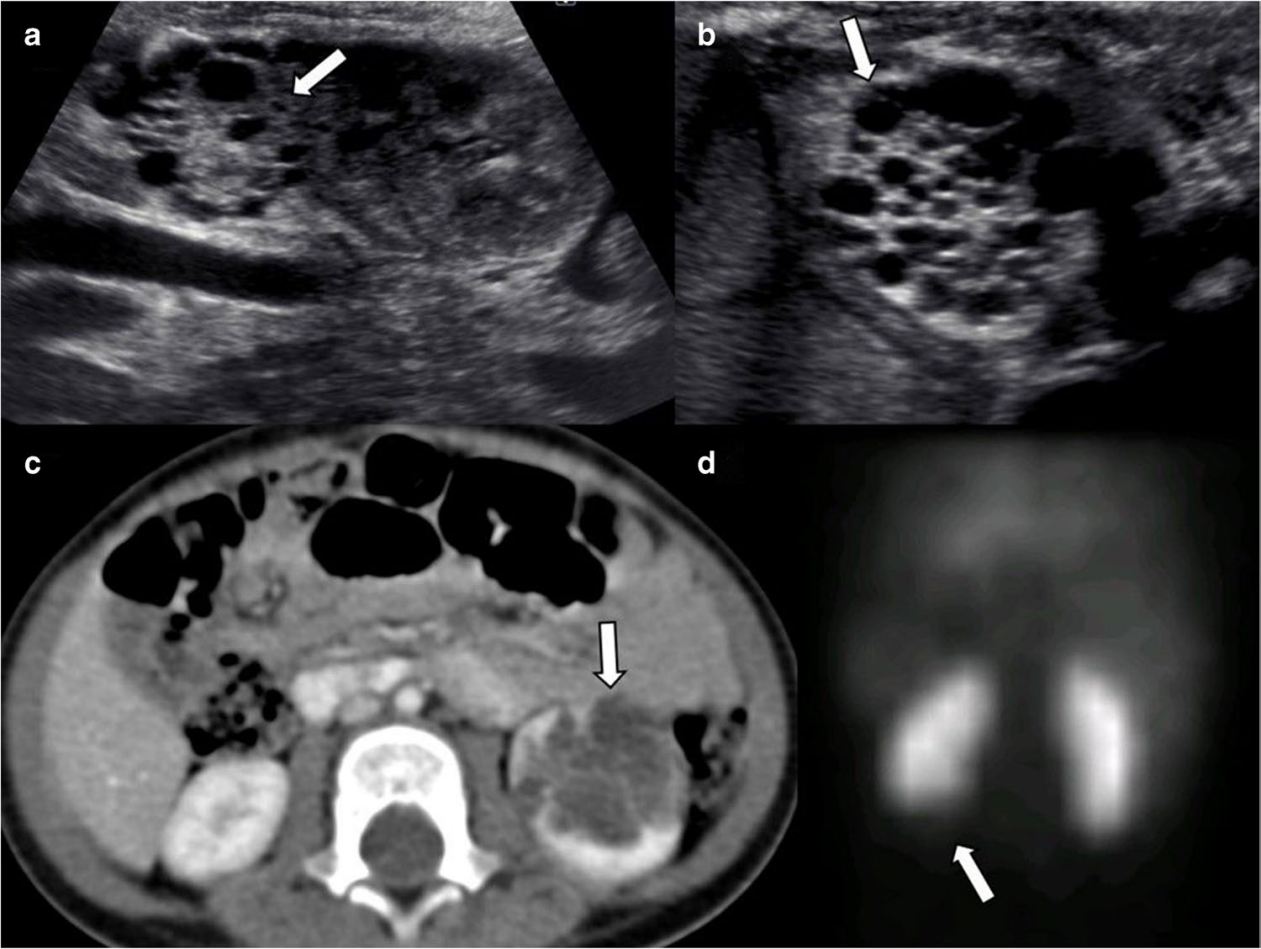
Segmental multicystic dysplastic kidney. Two girls aged 3 months (a, b) and 15 months (c, d) presented to the hospital with recurrent urinary tract infections. a, b Longitudinal (a) and axial (b) grayscale ultrasound images show a lesion (*arrows*) composed of non-communicating small cysts and echogenic stroma in the upper pole of the left kidney. c Axial contrast-enhanced computed tomography image reveals a multilocular cystic lesion composed of cysts of various sizes (*arrow*) in the lower pole of the left kidney. d Dimercaptosuccinic acid scintigraphy shows an absence of radiotracer uptake at the corresponding level (*arrow*). These lesions remained stable during follow-up
